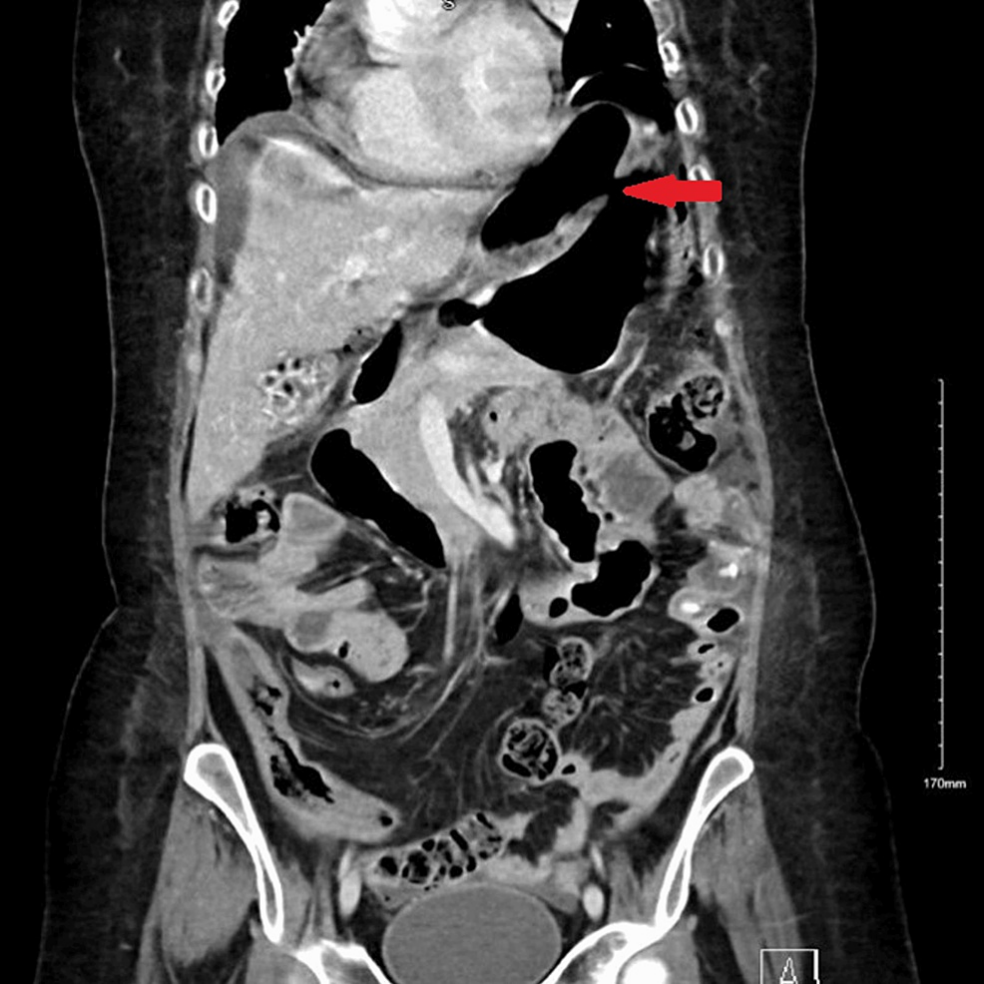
Coronal-section CT image of abdomen and pelvis at the time of gastric perforation showing features of gastric perforation likely related to the underlying perforated peptic ulcer with 0.8 cm defect at the distal greater curvature
